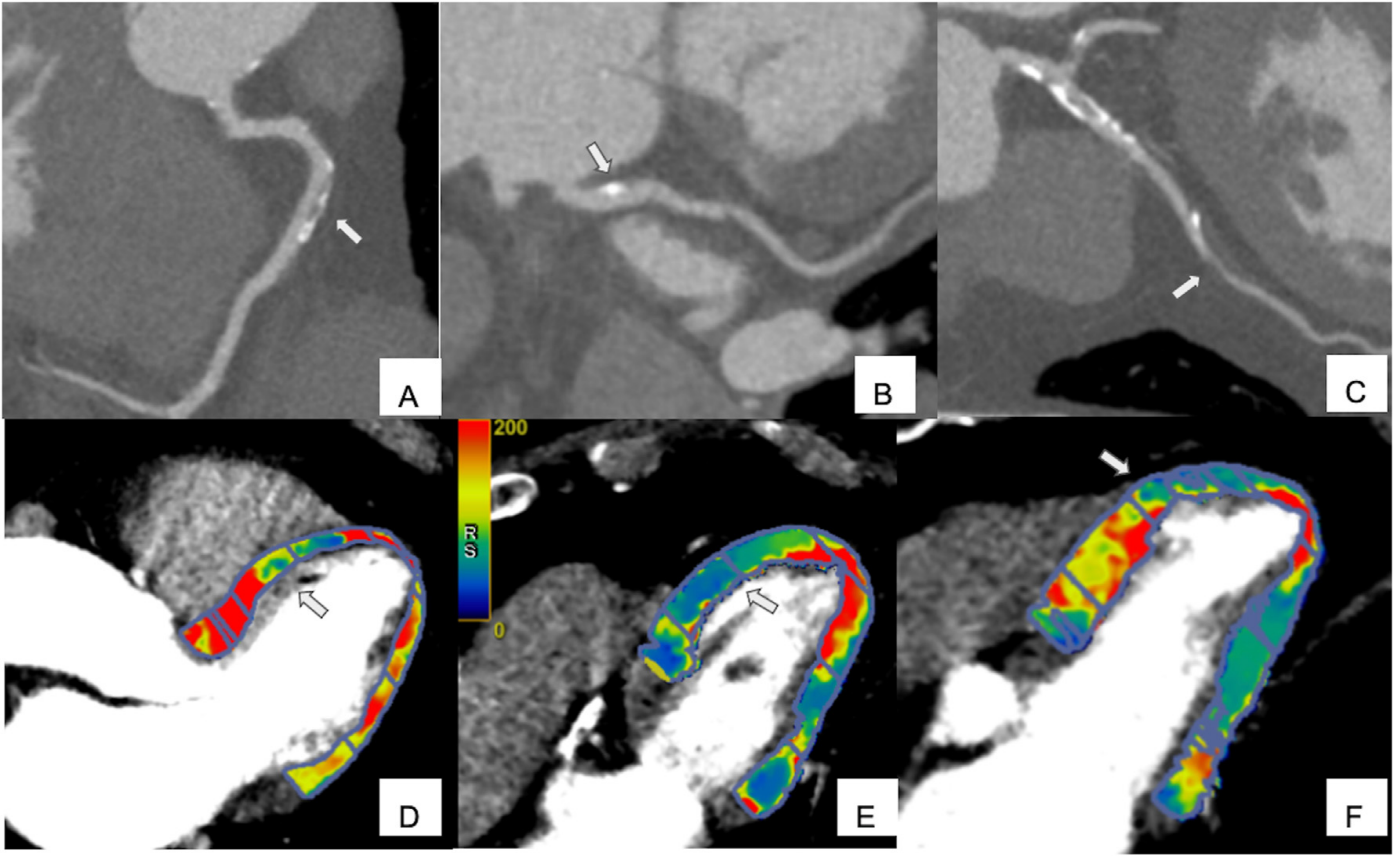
81-year-old male patient underwent perfusion CT for atypical pain and dispnea. Right coronary artery (A, arrow) and left circumflex (B, arrow) shows mild mixed plaque while left anterior descending artery demonstrates severe fibrofatty stenosis (C, arrow). The findings are then confirmed by the perfusion study, which shows antero septal mid-ventricle (D, arrow) and anterior mid-ventricle (E, arrow) and anterior apical segment (F, arrow).
